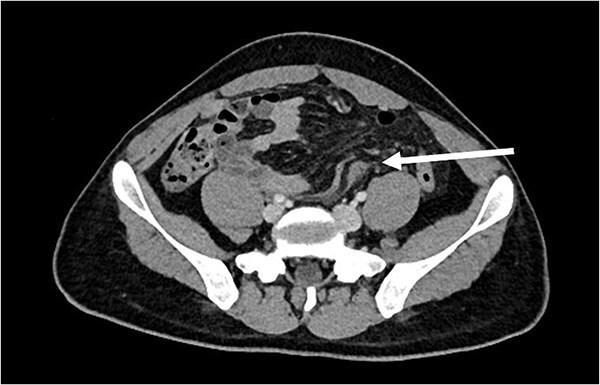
Axial CT image showing the thick band near the iliac vessels (arrow).
